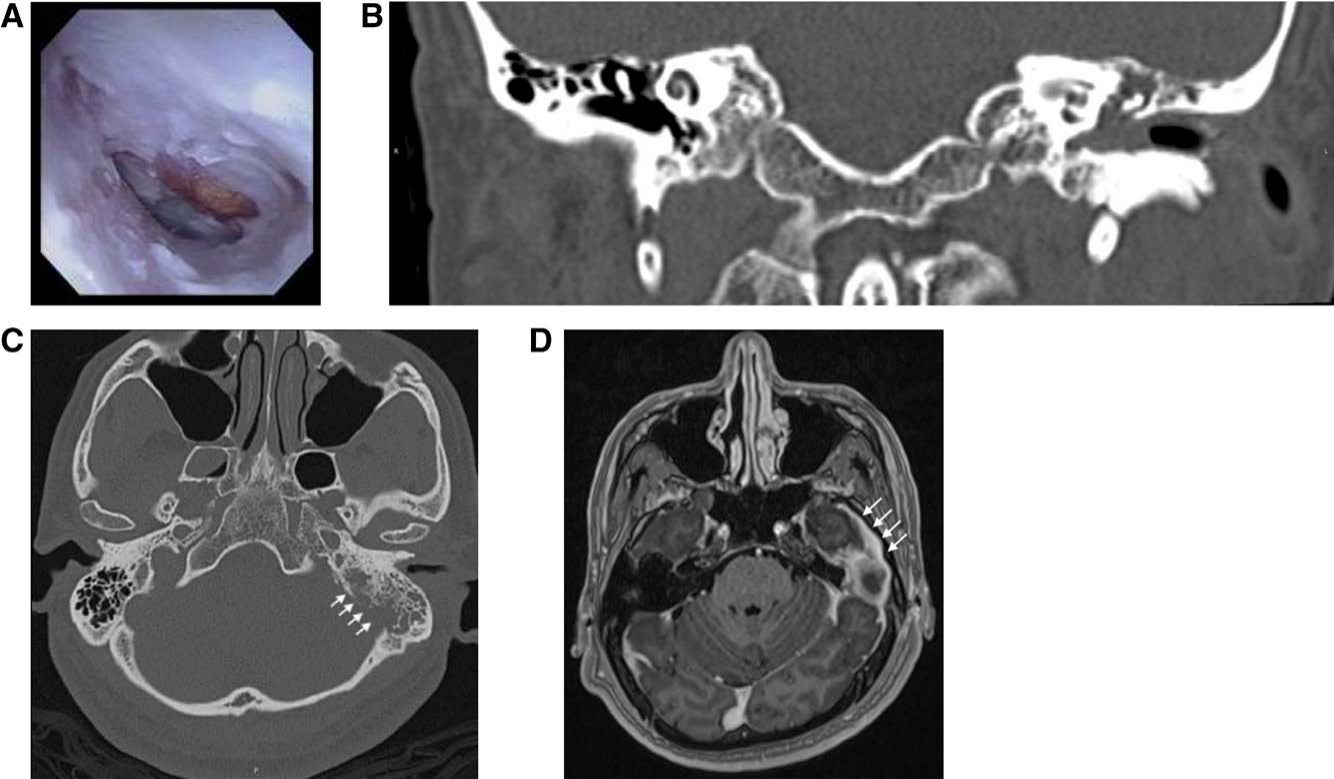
(A) Otoscopic preoperative evaluation, granulations at the attic, and posterosuperior quadrant. (B,C) CT temporal bone, (B) axial and (C) coronal view, showing initial left cavity and soft tissue lesion, and recurrent erosions of mastoid (white solid arrow). (D) MRI showed left otomastoditis with subtle meningitis. CT = computed tomography, MRI = magnetic resonance imaging.
